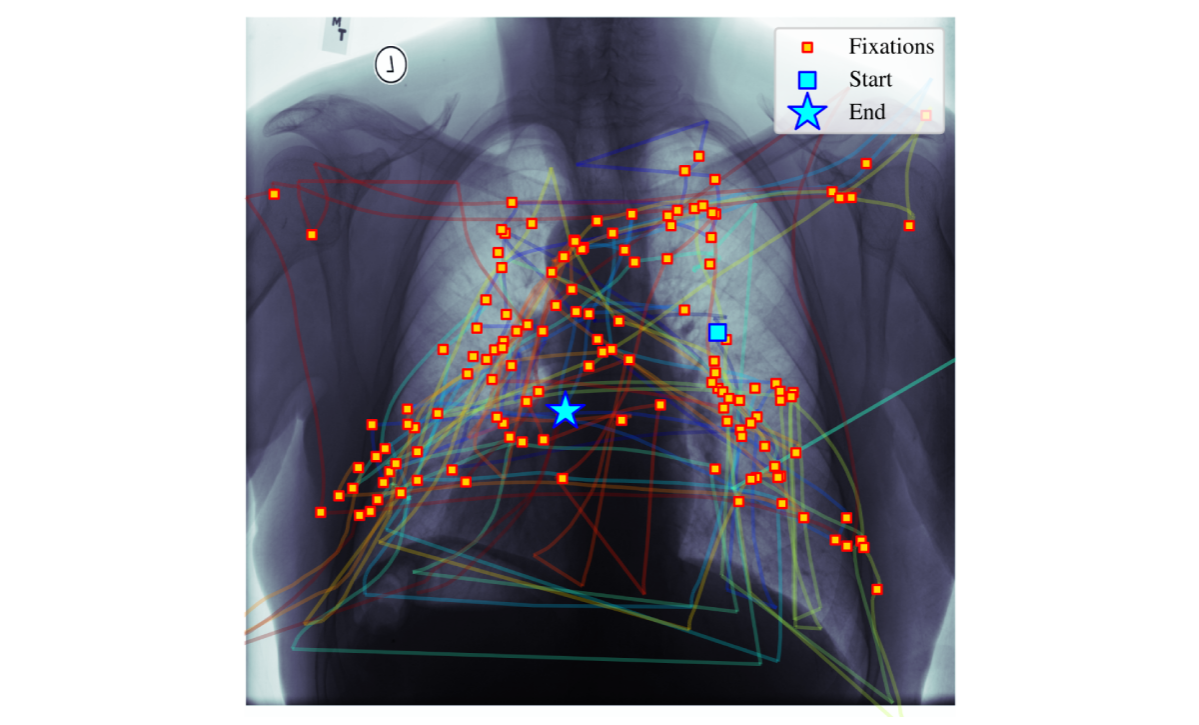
Scan of chest x-ray by participant 2 (trainee).
